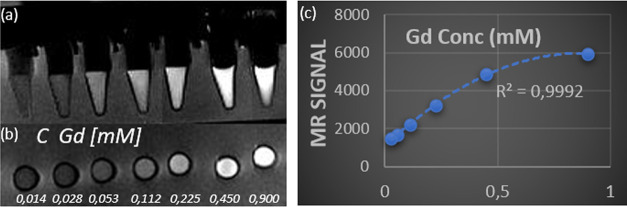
T*_1_-weighted MR images of CeO*_x_*:Gd9%
